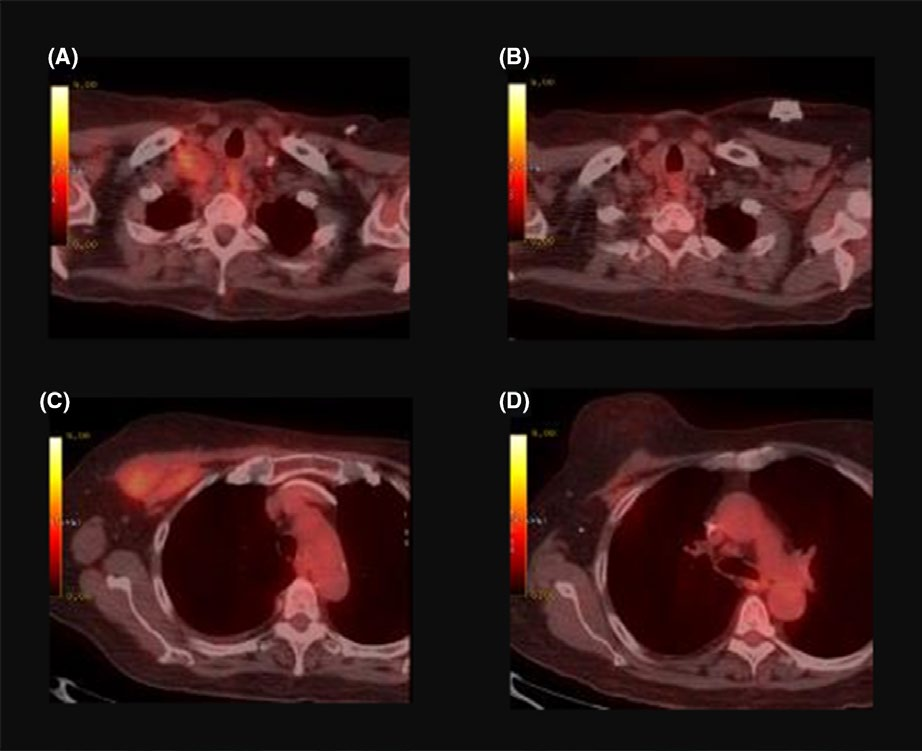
PET/CT images demonstrating the treatment response to brentuximab vedotin. A, Pretreatment hypermetabolic supraclavicular adenopathy. B, Resolution of supraclavicular adenopathy after the 5th cycle of BV. C, Hypermetabolic mass in the right anterior chest wall. D, Resolution of the mass after the 5th cycle of BV with formation of a large seroma (not shown)
